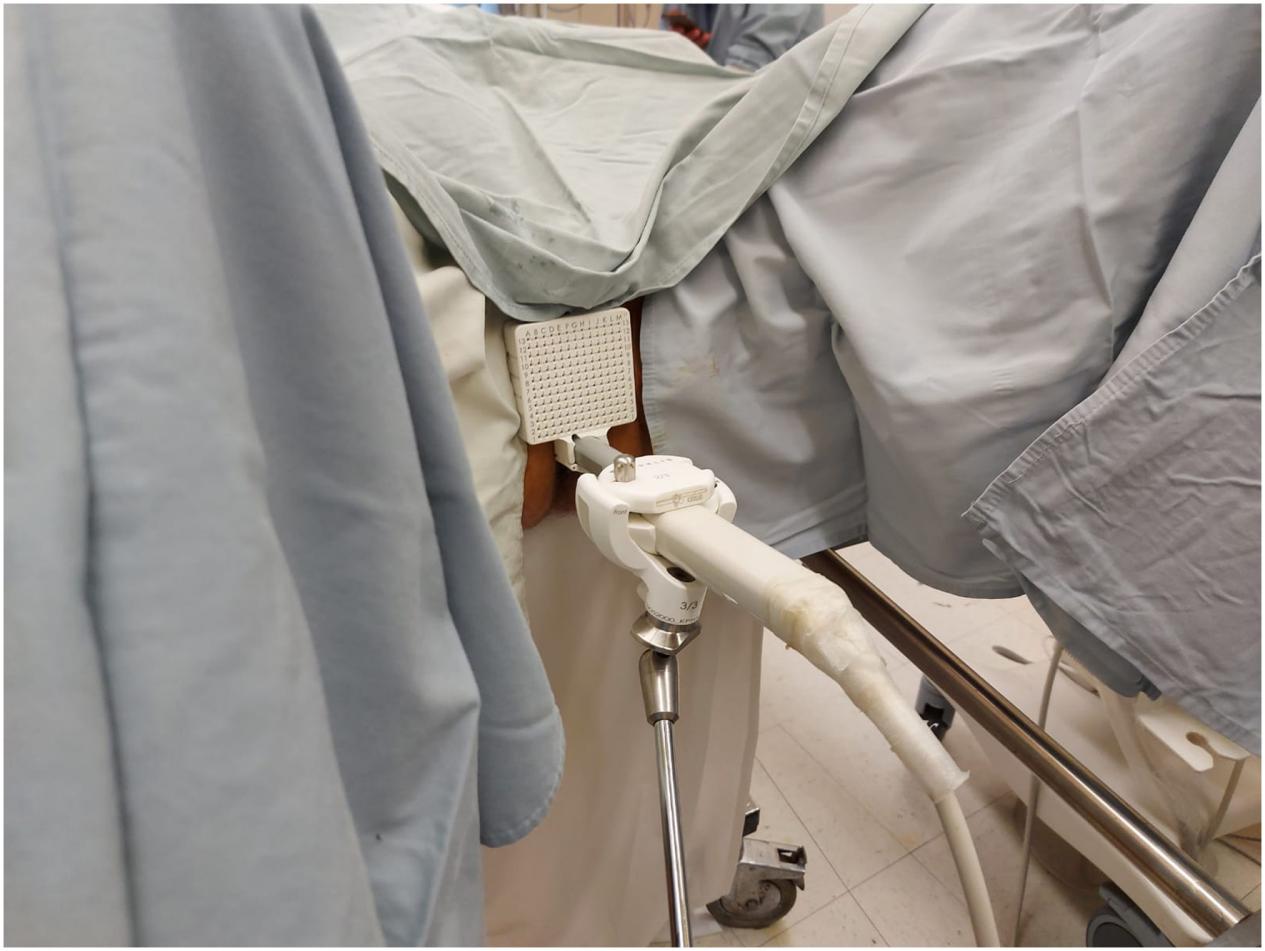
Patient prepped and draped in the lithotomy position. US probe and grid mounted on a stable stepper. US, ultrasound.
